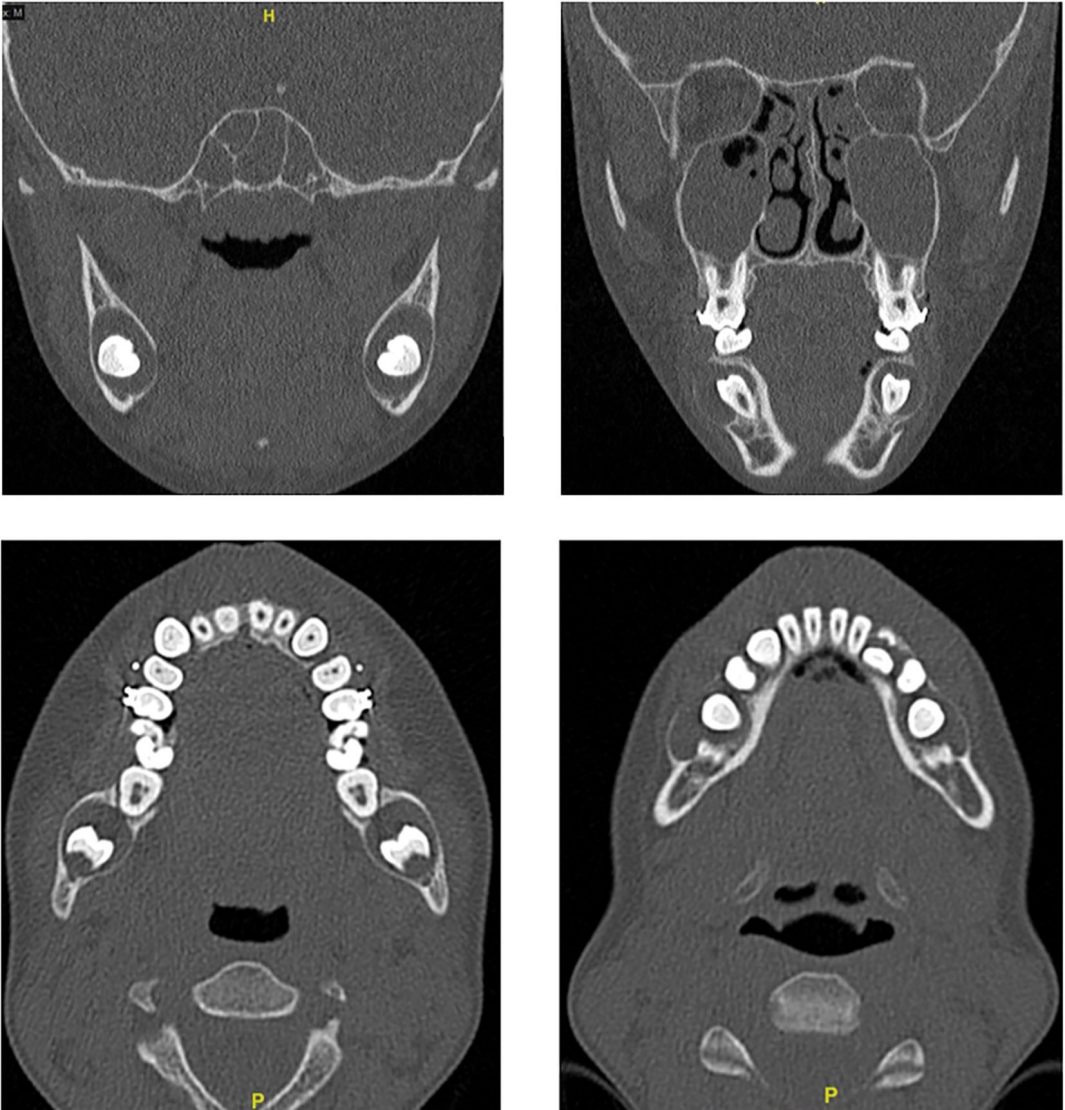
Preoperative CBCT showing unerupted teeth and bone expansion in coronal and axial views.
